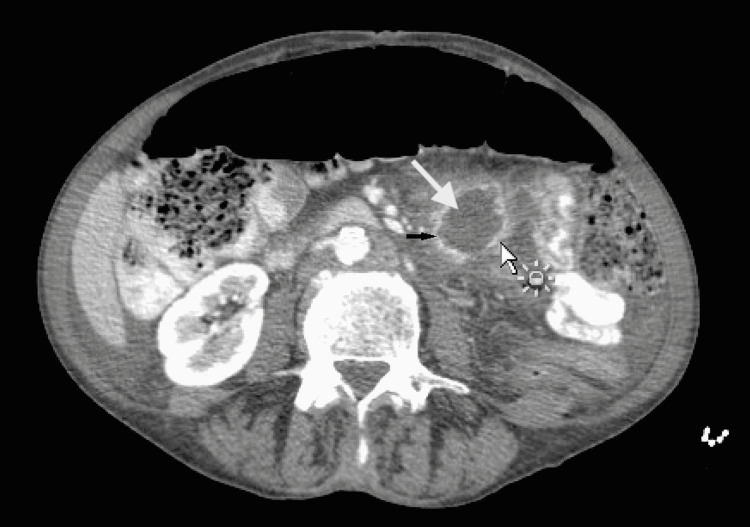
CT of the abdomen and pelvis with contrast showing 3.2 x 3.8 x 2.7 cm irregularly marginated peripherally enhancing fluid collection in the left mid abdominal mesentery with prominent surrounding inflammation consistent with abscess. A white short arrow shows surrounding inflammation, a white large arrow shows necrotic material, and a black arrow shows ring enhancement.
